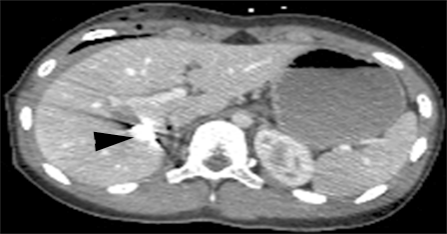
Abdominal CT. Black arrow – segment Ⅴ metallic fragment.
